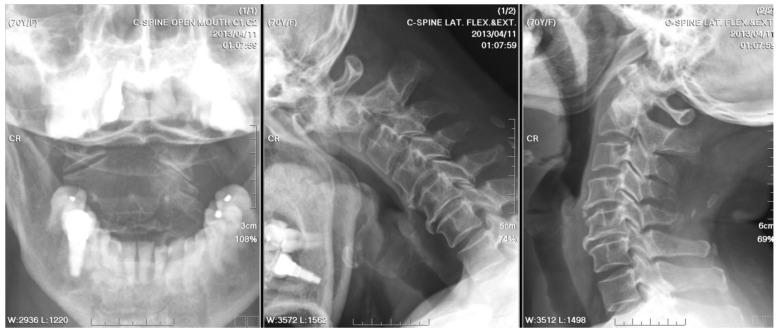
Initial radiographs of the cervical spine: They identified bony destruction of the C2 odontoid process and atlantoaxial subluxation.
